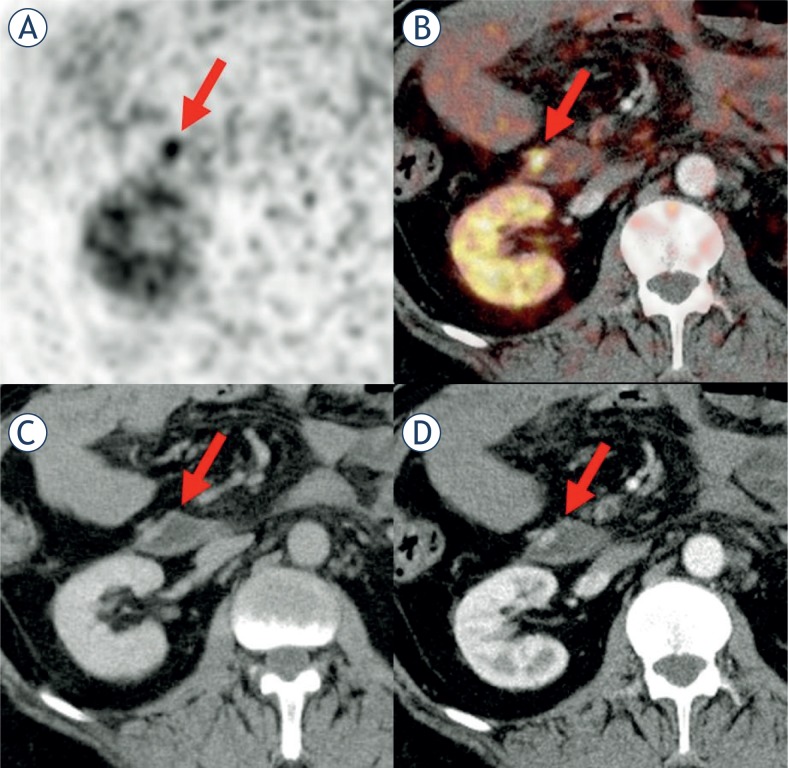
Another suspicious Ga-68-DOTATOC PET lesion (#11) (A) located in the duodenum (B) without a clear correlate in the venous-phase CT (C), but arterial hyperperfusion (D).
